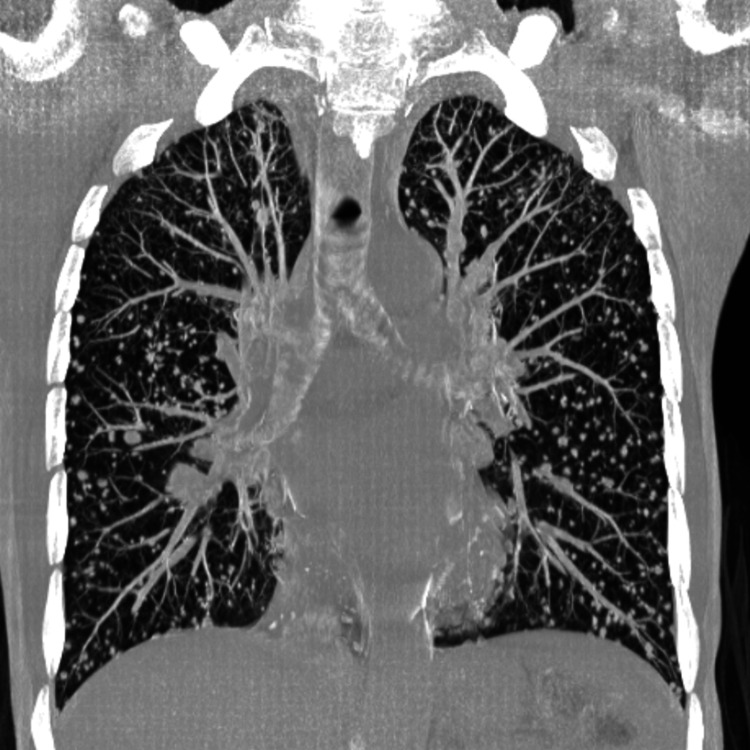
Thoracic CT in the lung window (coronal plane, MIP reconstruction) shows multiple pulmonary nodules and micronodules of varying shape and size, consistent with metastatic disease CT: computed tomography; MIP: maximum intensity projection
